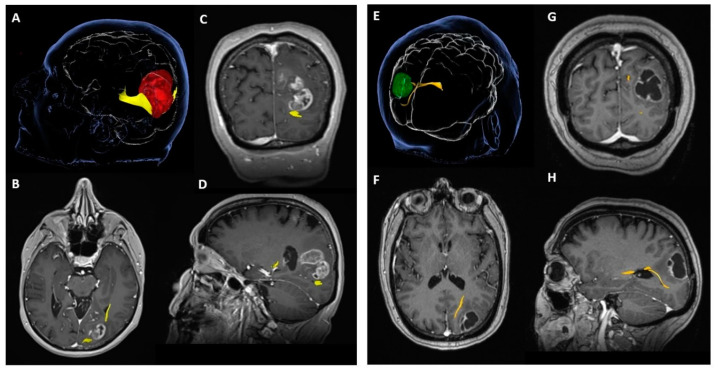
Example of two different tumours in the visual eloquent areas of the brain. (A) Probabilistic tractography of the optic radiation, infiltrated by the tumour (tumour—red, optic radiation—yellow); (B) axial T1-weightened MRI image post-gadolinium; (C) coronal view; (D) sagittal view; (E) probabilistic tractography of the optic radiation, displaced by the tumour without infiltration (tumour—green, optic radiation—yellow); (F) axial T1-weighted MRI image post-gadolinium; (G) coronal view; (H) sagittal view.
